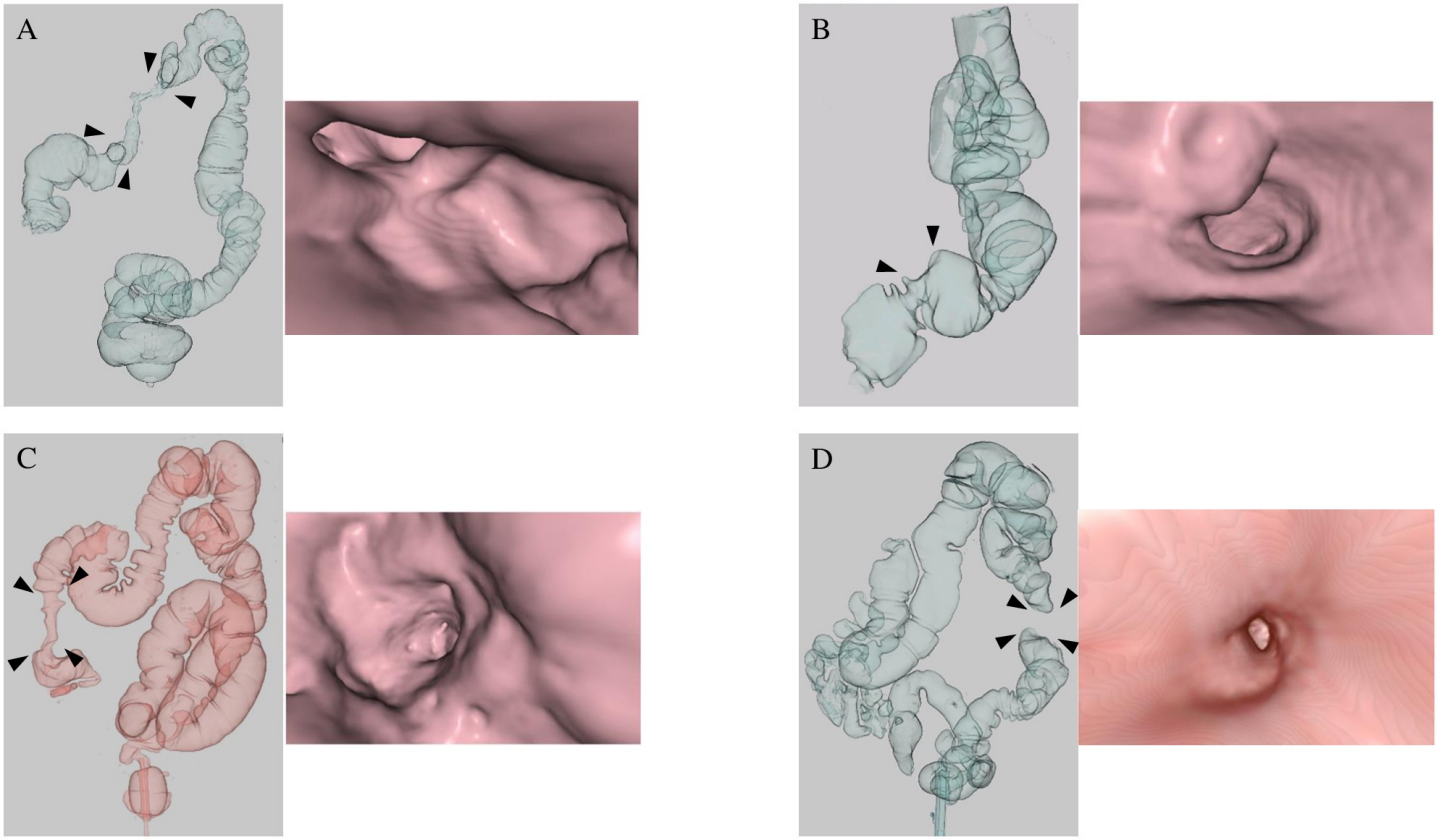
Representative computed tomography colonography (CTC) images.
